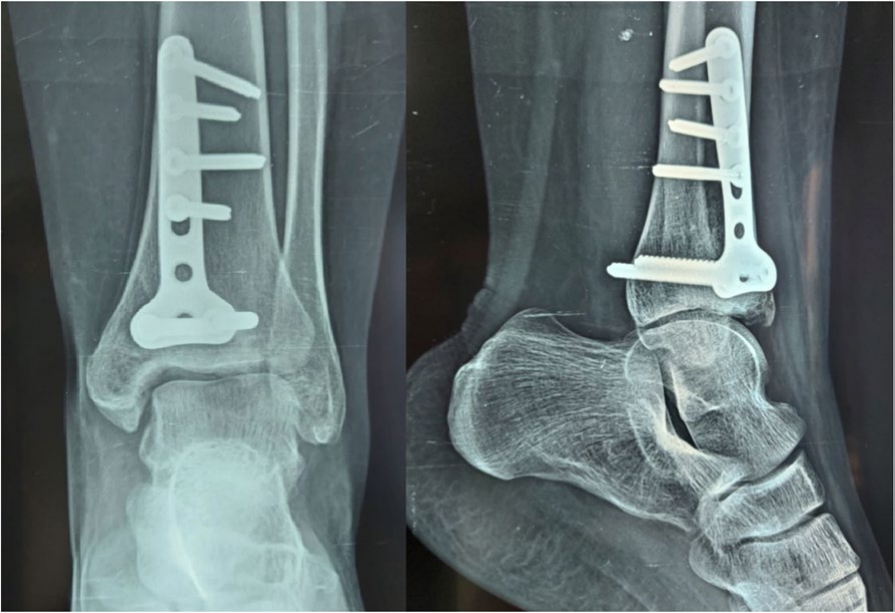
Fifteen months anteroposterior and lateral plain X-rays showing no signs of local recurrence
